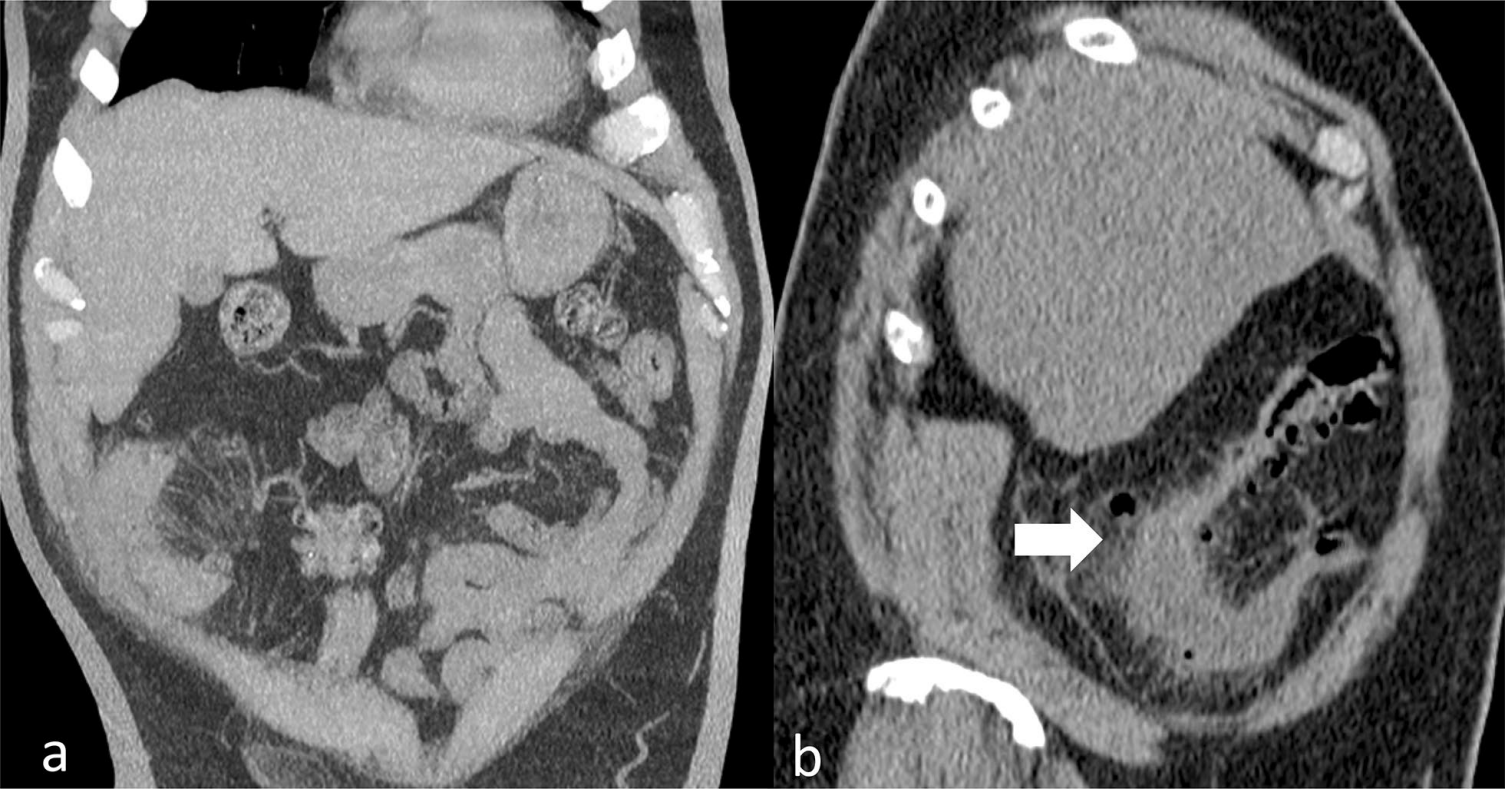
The CT scan of the abdomen and pelvis without intravenous contrast material. In a, the coronal reformat and in b the sagittal reformat. The scan showed the wall thickening of the left and sigmoid colon, with diverticular outpouching. Moreover, there was stranding of the perivisceral fat due to perivisceral tissue inflammation complicated with limited perforation and a small free air pocket next it; therefore, the diagnosis favoured was diverticulitis with limited perforation (white arrow). There was no free fluid
